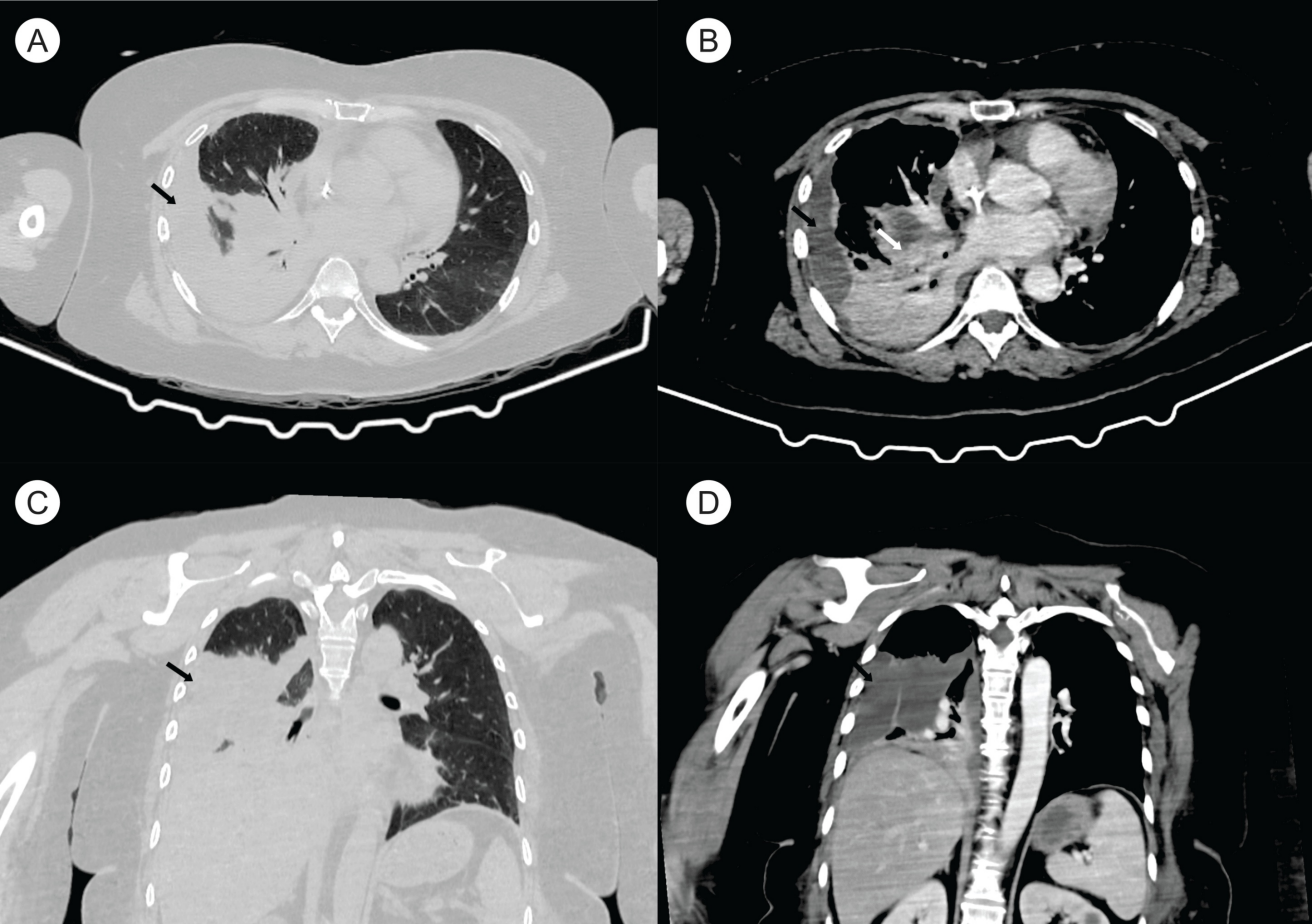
Chest CT done on admission showing a right pleural effusion Pleural effusion (black arrows in A-D) and a consolidative lesion (white arrow in B) are seen in the right lung. (A) Axial chest CT with lung window. (B) Axial chest CT with mediastinal window. (C) Coronal view with lung window. (D) Coronal view with mediastinal window.
